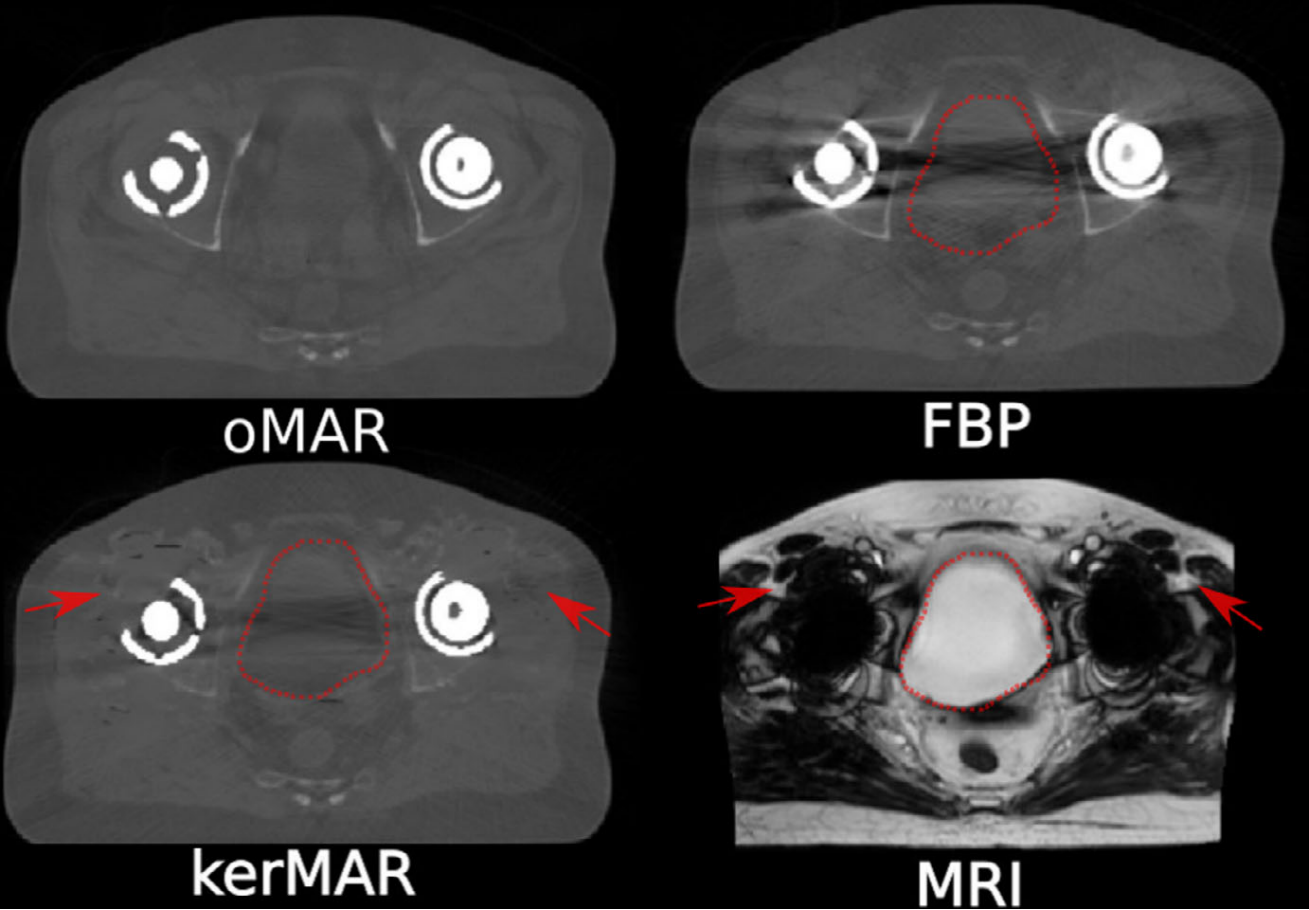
The Philips metal artifact reduction for orthopedic implants (oMAR), the filtered back projection (FBP), our magnetic resonance (MR)‐based kernel regression metal artifact reduction (kerMAR) and the T1w MR image (MRI) of a pelvis with metallic dual hip implants. The delineation of the bladder was performed in the MR image. The arrows point to regions where metal artifacts on the MRI led to artifacts in the kerMAR. [Color figure can be viewed at http://www.wileyonlinelibrary.com/]
